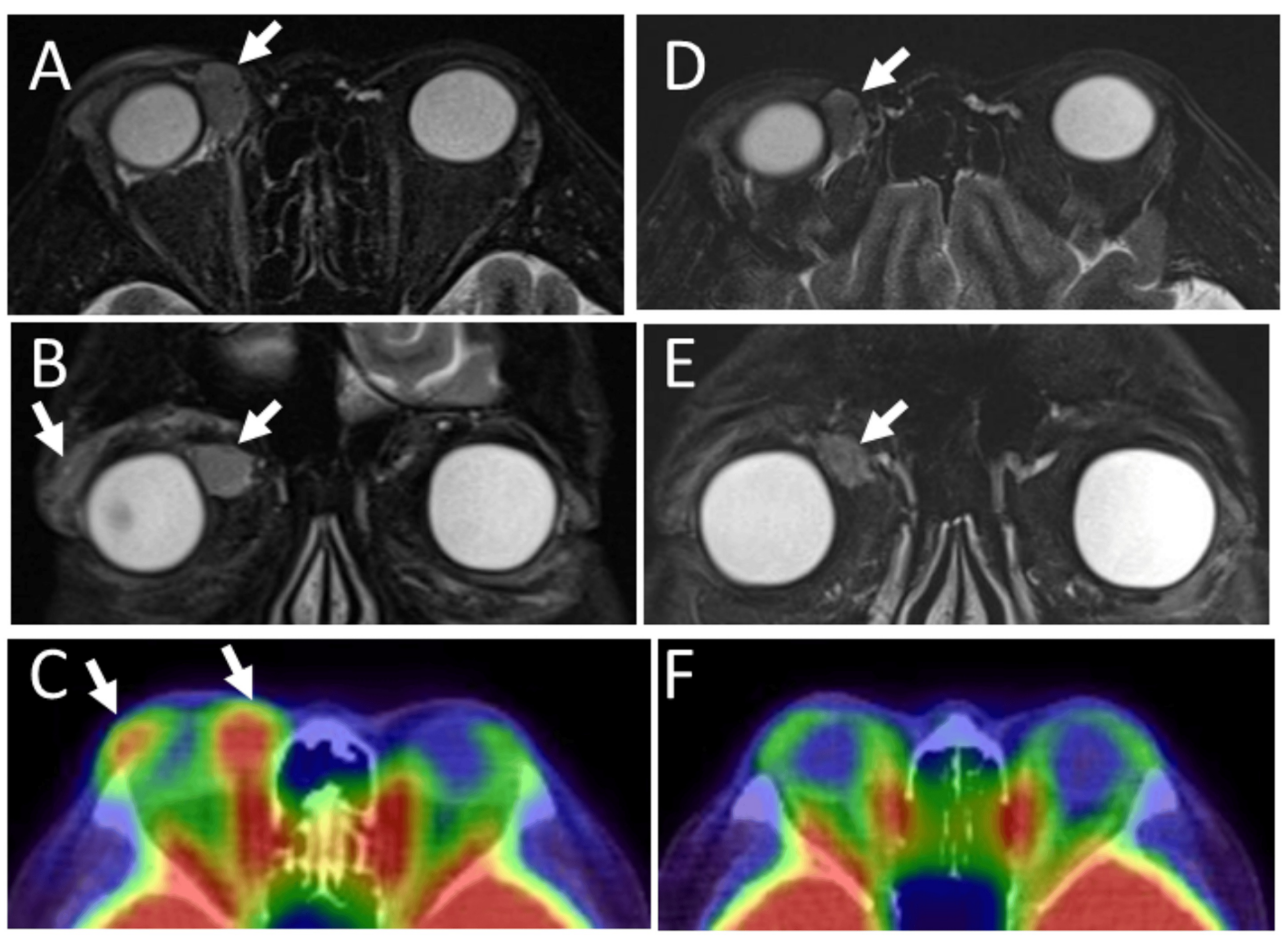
Magnetic resonance imaging and positron emission tomography at ages 54 and 55 T2-weighted STIR (short tau inversion recovery) magnetic resonance imaging at age 54, 10 months after left orbital mass excision, showing an enlarged lacrimal gland mass and a new nasal-superior mass (arrows, A, B) in the right orbit and no lesion in the left orbit (A: axial image, B: coronal image). Fluorodeoxyglucose positron emission tomography (C), showing high uptake sites (arrows) in the lacrimal gland and the nasal mass in the right orbit. T2-weighted STIR magnetic resonance imaging after four injections of weekly rituximab monotherapy in a month (D: axial image, E: coronal image). Note the diminished mass in the right nasal orbit (arrows, D, E) and no lesions in the bilateral lacrimal glands. Positron emission tomography (F) 7 months after rituximab monotherapy, showing no abnormal uptake in bilateral orbits.
